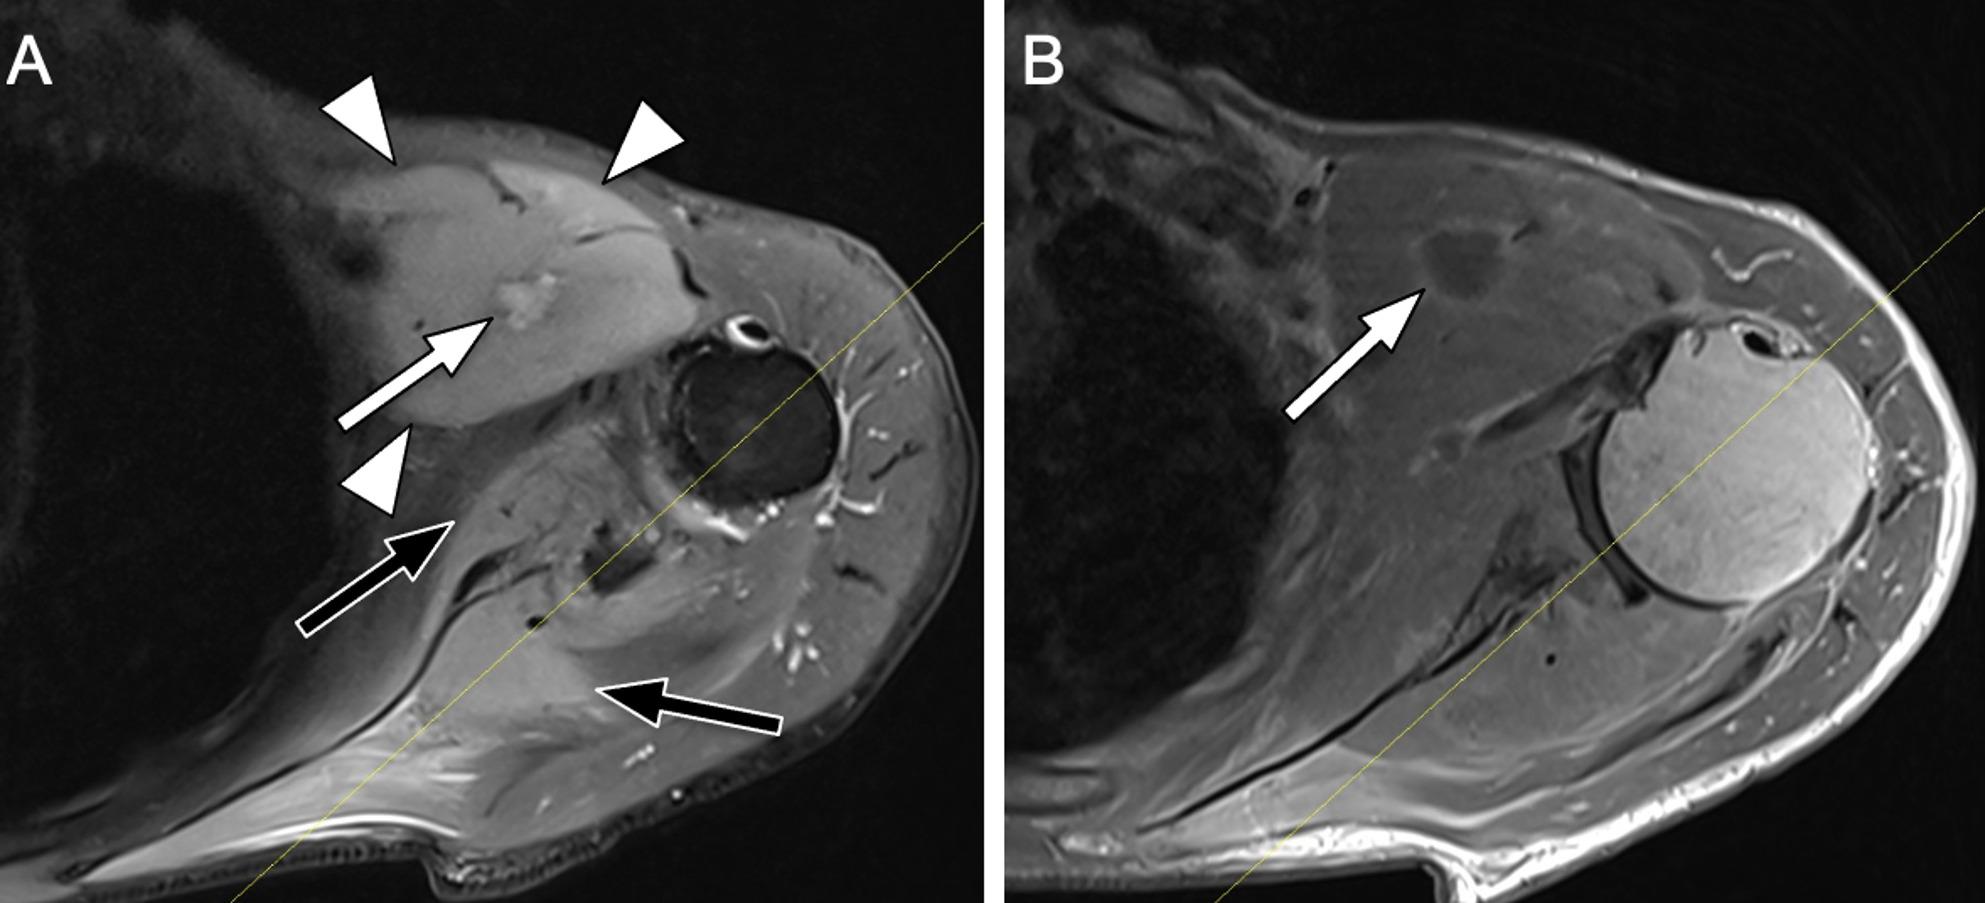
MRI of an 85-year-old female presenting with non-Hodgkin lymphoma of the shoulder. A Transversal PD-weighted image shows a hyperintense tumor (arrowheads), involving m. deltoideus, m. pectoralis major, m. infraspinatus and m. subscapularis (black arrows) and central signal alteration (white arrow). B Transversal contrast enhanced T1-weighted image reveals central necrosis (arrow)
